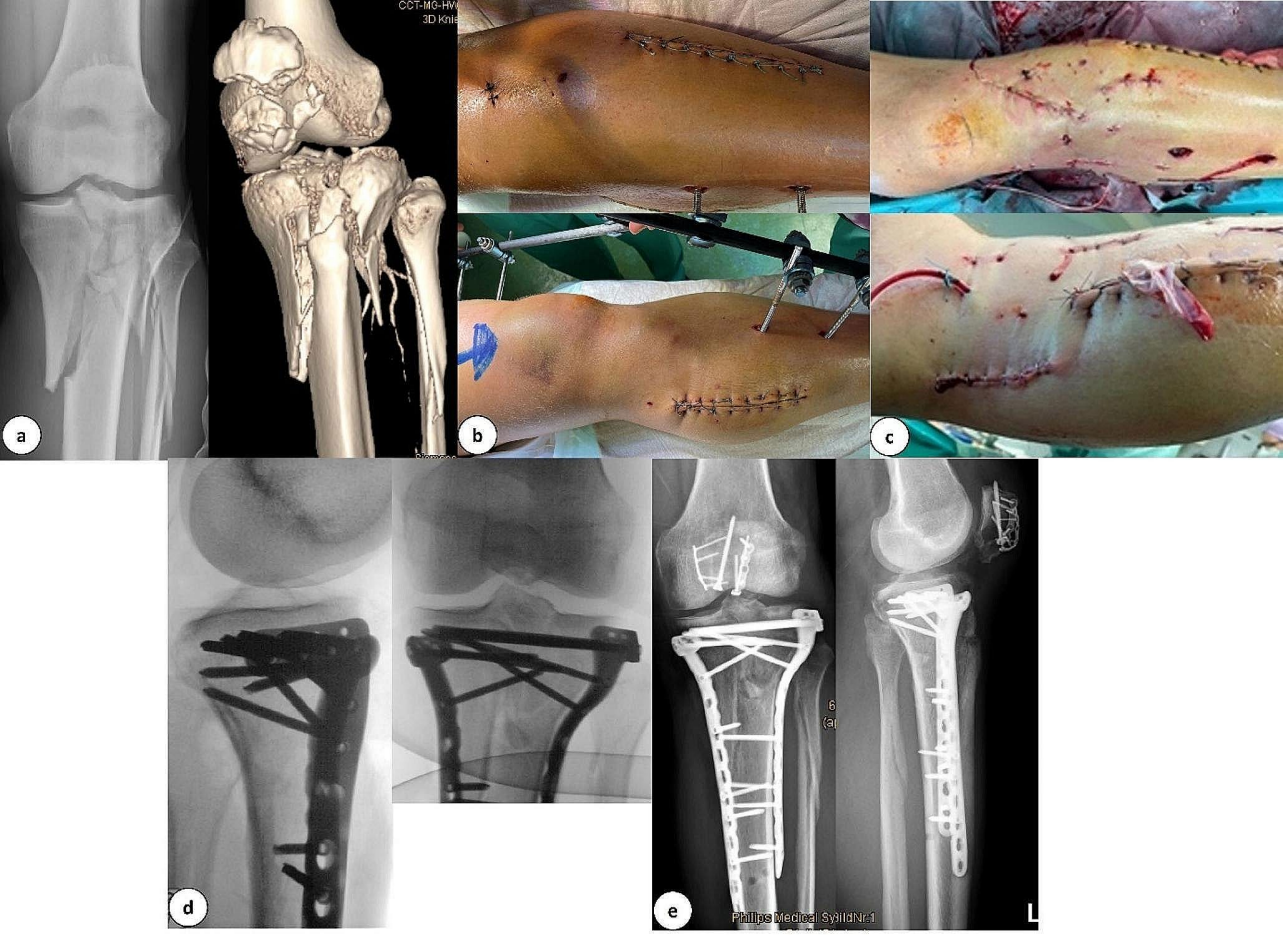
a: Anterior-posterior X-ray and 3-D computed tomography scan of a tibial plateau fracture (AO: 33C3.3). b: Preoperative clinical images before minimally invasive double plate osteosynthesis with a knee joint bridging external fixator. A condition after compartment release. c: Clinical images after minimally invasive double plate osteosynthesis and wound closure. d: Intraoperative final X-ray in 2 planes with double plate osteosynthesis. e: X-ray control 10 days postoperatively with double plate osteosynthesis in the area of the tibial head and miniplate with screw osteosynthesis of the patella
